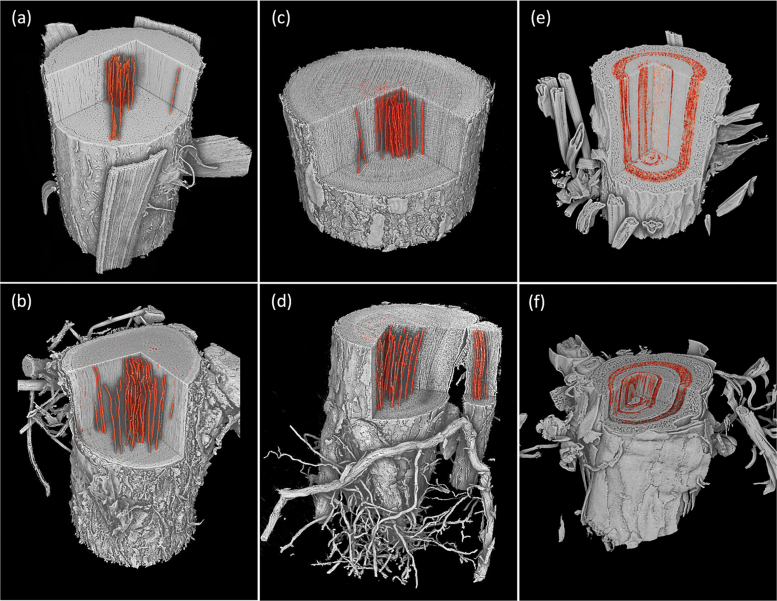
Representative images showing rendering of stem and root volumes visualized using X-ray microtomography from one individual of *Acacia aneura* (A and B), *Eucalyptus saligna* (C and D), and *Cedrus deodara* (E and F). Cut away in root and stem volumes shows the internal anatomy of the plant with embolized vessels or tracheids highlighted in orange. Cut away sections in root and stem volumes show the internal anatomy of the plant, with embolized vessels rendered in orange for angiosperm species *A. aneura* and *E. saligna*. In stems and roots of the conifer species *C. deodara*, the area of embolized xylem tracheids is shown in orange. The field of view for scans was 28 mm in *X* and *Y* orientations and 20 mm in *Z* (vertical) orientation for stems and roots. A sub-volume of scans has been rendered in each panel. (This figure is available in colour at JXB online.)
